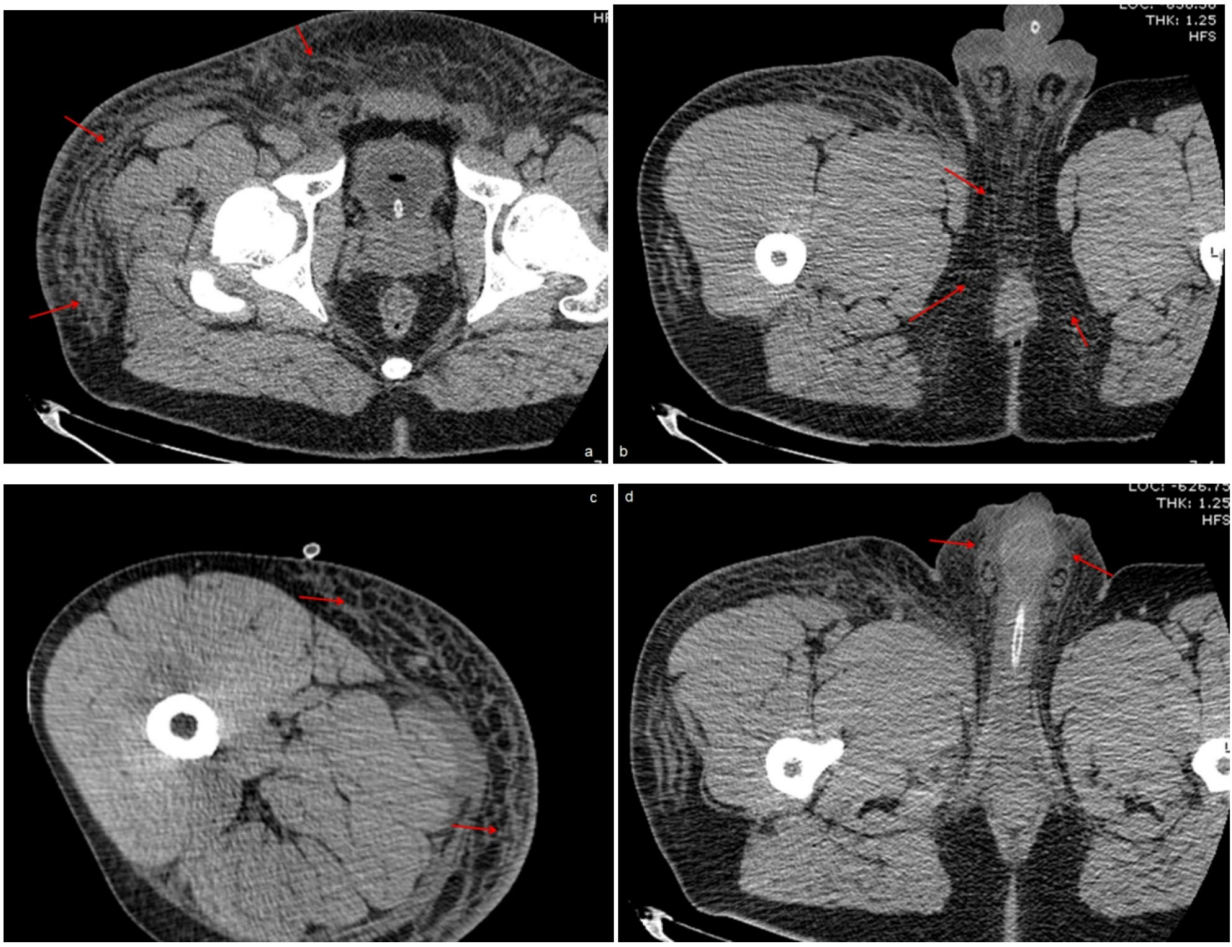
CT imaging showing subcutaneous soft tissue edema in the right hip, pelvic floor, and medialright thigh
